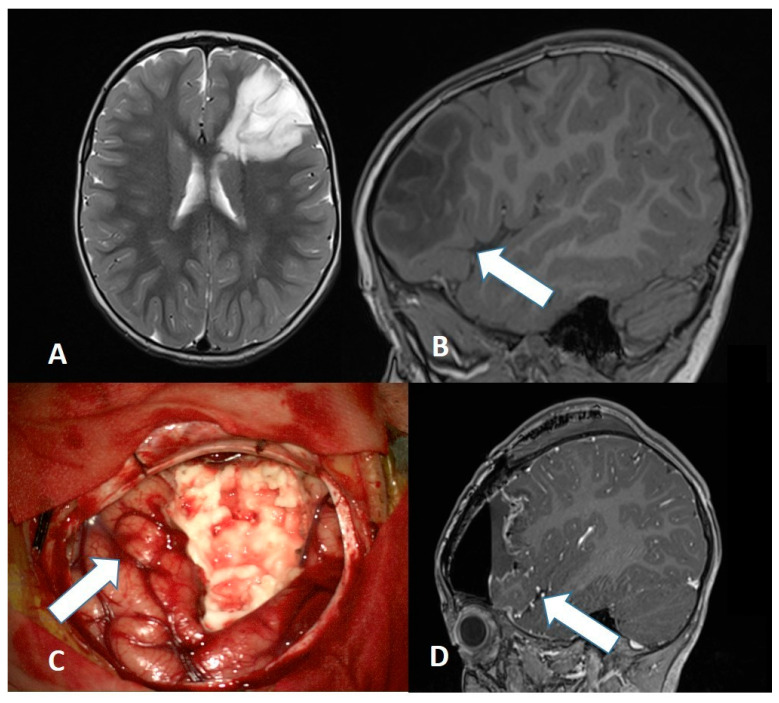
Management of a benign dysembryoplastic neuroepithelial tumor (DNET) (A,B) involving the Broca area (white arrow). While awake surgery was unfeasible due to the patient’s age, the eloquent speech area was preserved ((C), white arrow) using intraoperative neuronavigation, as confirmed by early postoperative MRI (white arrow indicates the anatomically preserved Broca area (D)).
